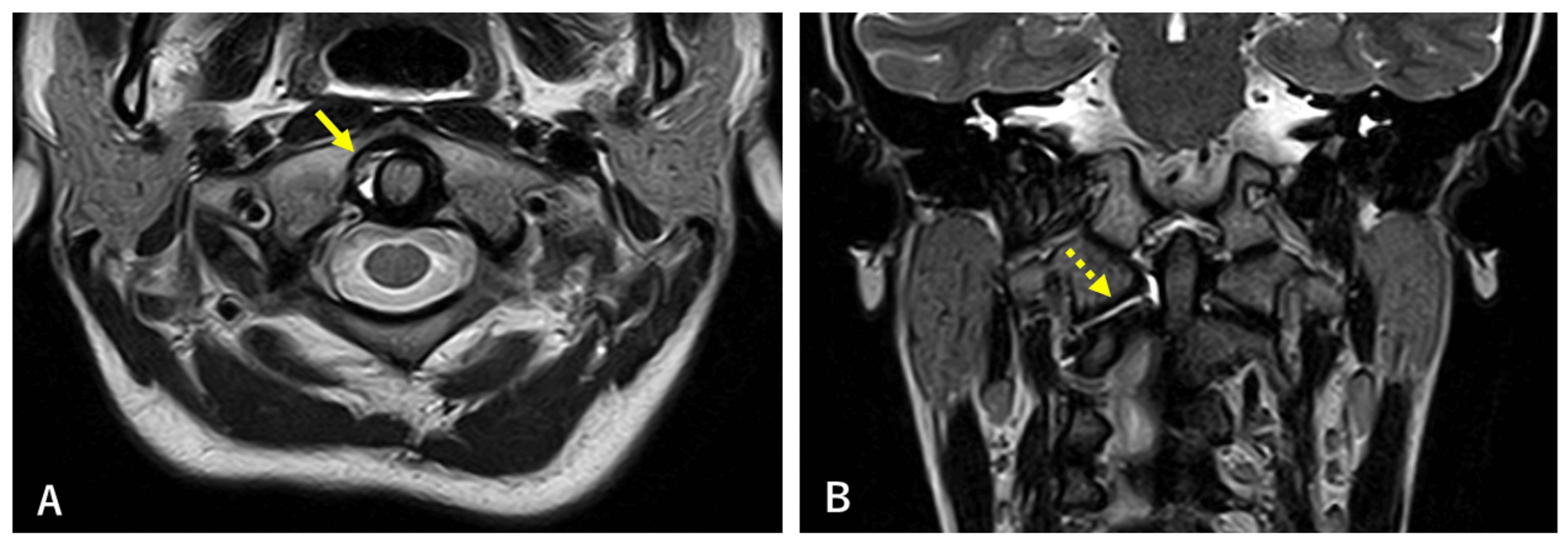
T2-weighted imaging of magnetic resonance imaging (MRI) There is a small amount of effusion on the right side of the odontoid (Figure 3a, arrow) and right facet joint (Figure 3b, broken arrow). No damage to the transverse ligament or alar ligament was observed.
